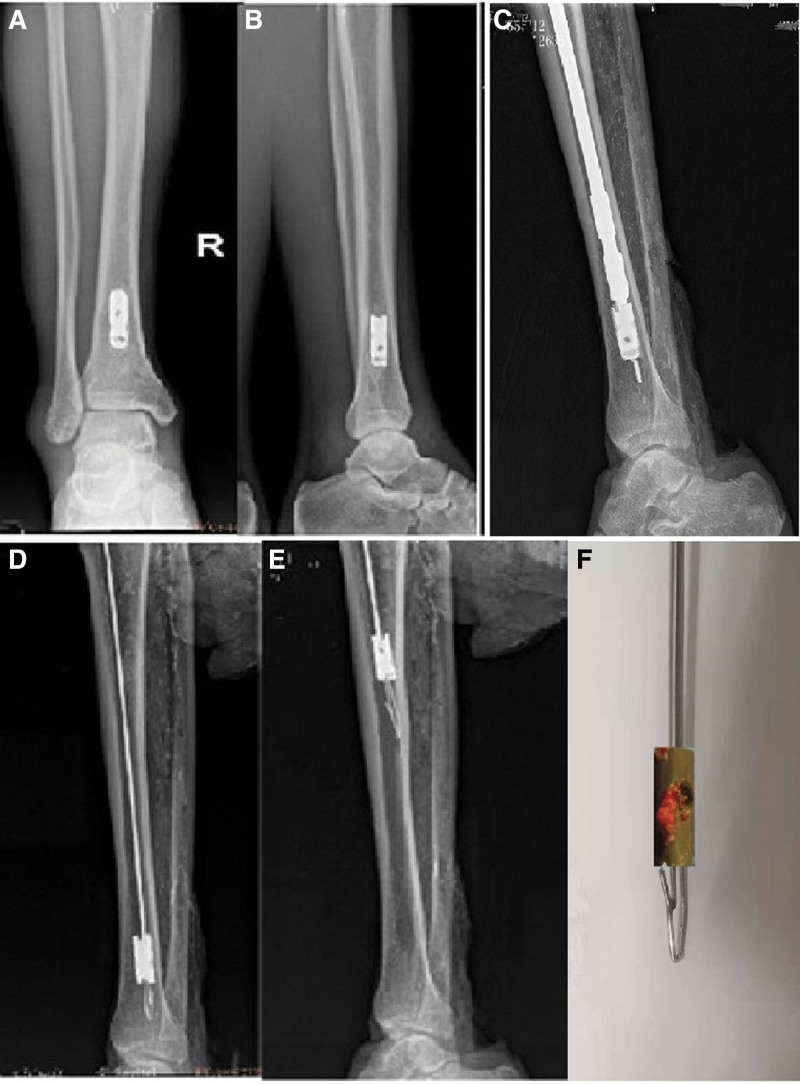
A 48-year-old male patient with fracture union and a distal broken segment of cannulated intramedullary nail. (A, B) X-ray showing fracture union and a distal broken end remaining at the tibial distal medullary cavity. (C) Intraoperative X-ray showing a drill reaming the medullary cavity along a guide needle. (D) Intraoperative X-ray showing that the broken nail extractor passed through the canal of the broken nail. (E) Intraoperative X-ray showing the barb of the broken nail hooking the canal wall. (F) The broken nail extractor extracted the broken nail.
